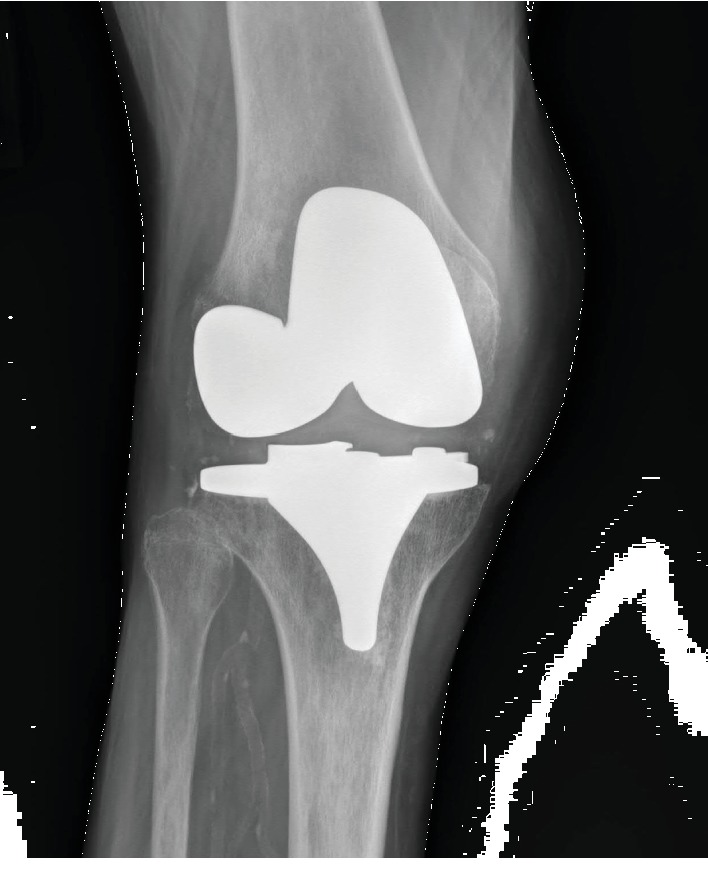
Anteroposterior radiograph of the right knee demonstrating TKA hardware without acute abnormality.
